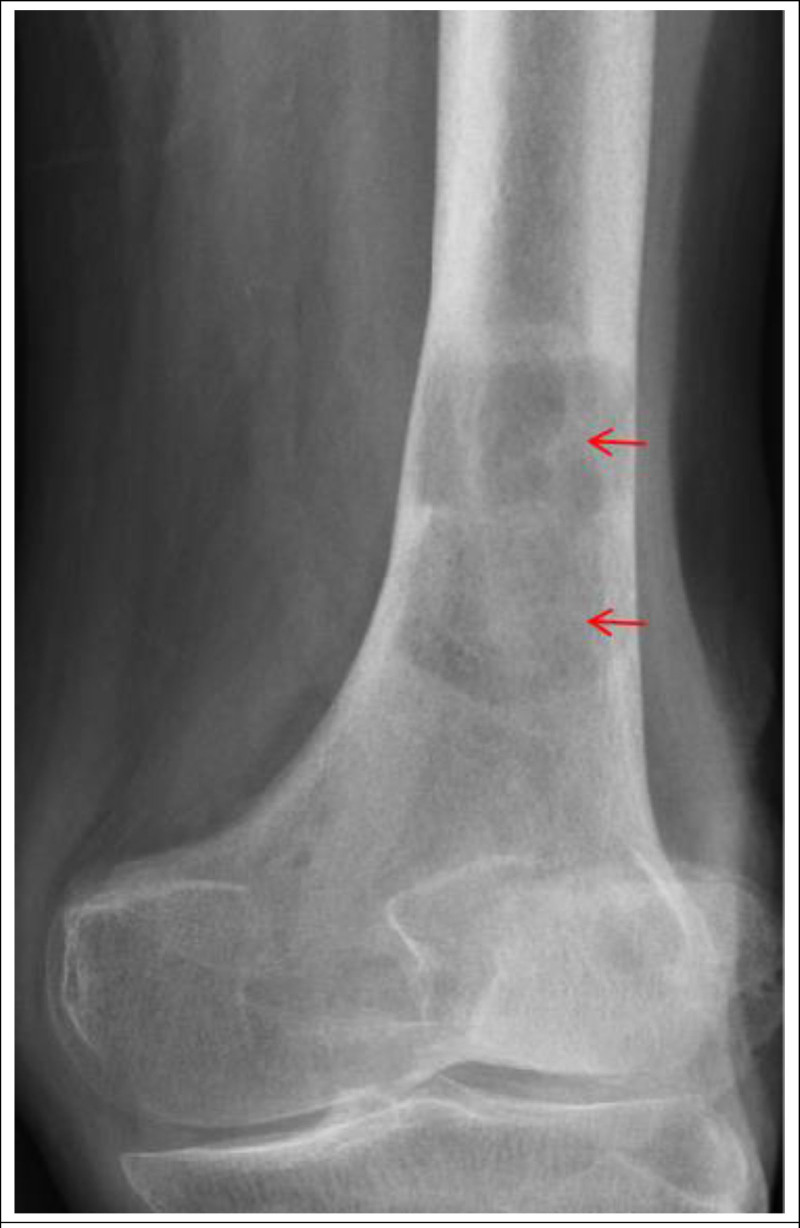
Radiographs of the right distal femur was also taken and showed bone lytic lesions (red arrows).
